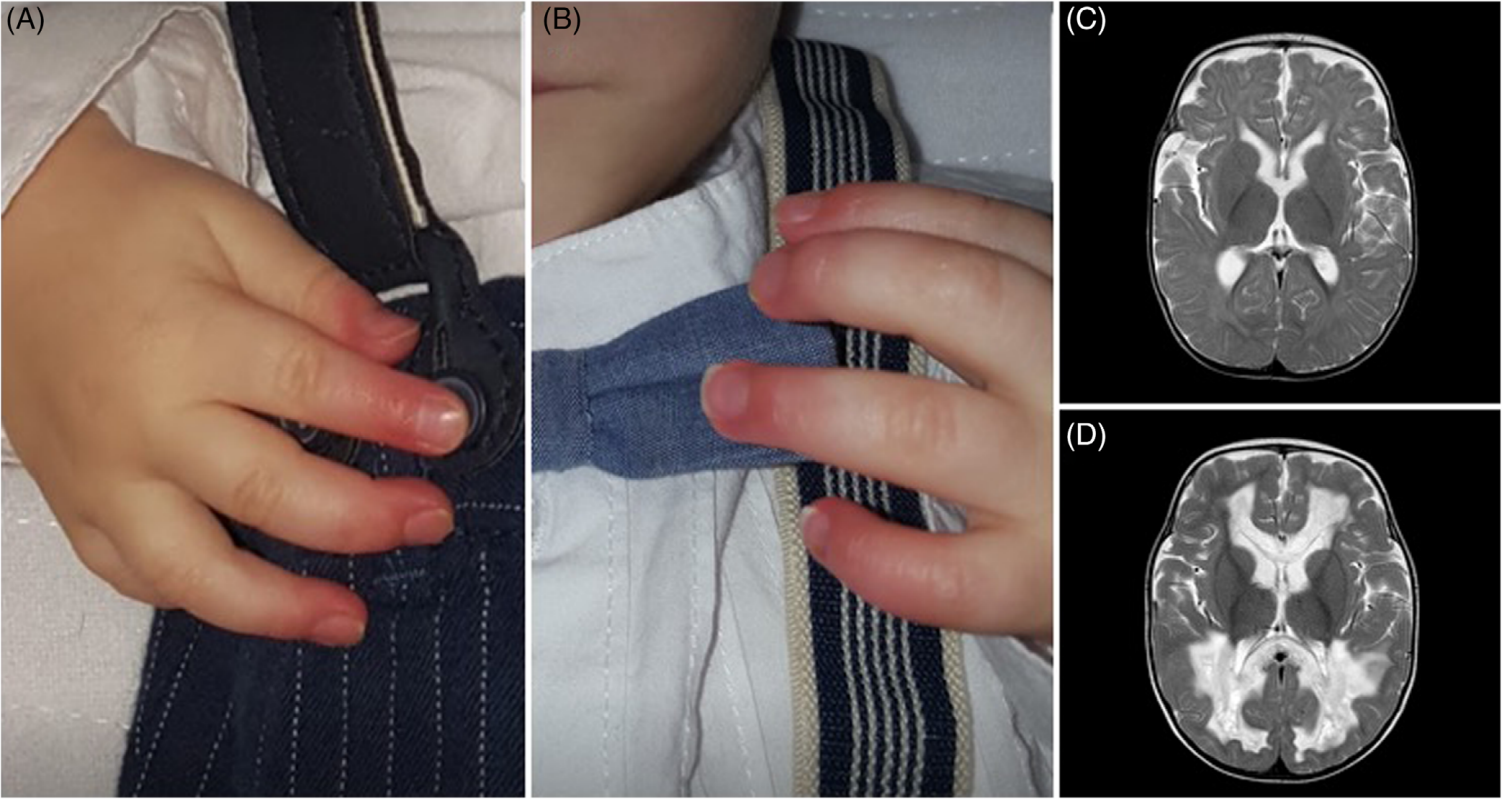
Finger findings and cerebral MRI in the reported patient. A and B, Chronic distal phalangeal erythema, an accompanying sign since the age of 5 months old. C, Axial T2W at the age of 12 months old showing mild cerebral atrophy. D, Axial T2W at the age of 24 months old with appearance of cystic change in white matter. White matter tracts surrounding the lateral ventricles and commissural fibres had signal abnormalities, showing small necrotic or porencephalic cysts. “U” fibres, pyramidal tracts and gray matter were spared
